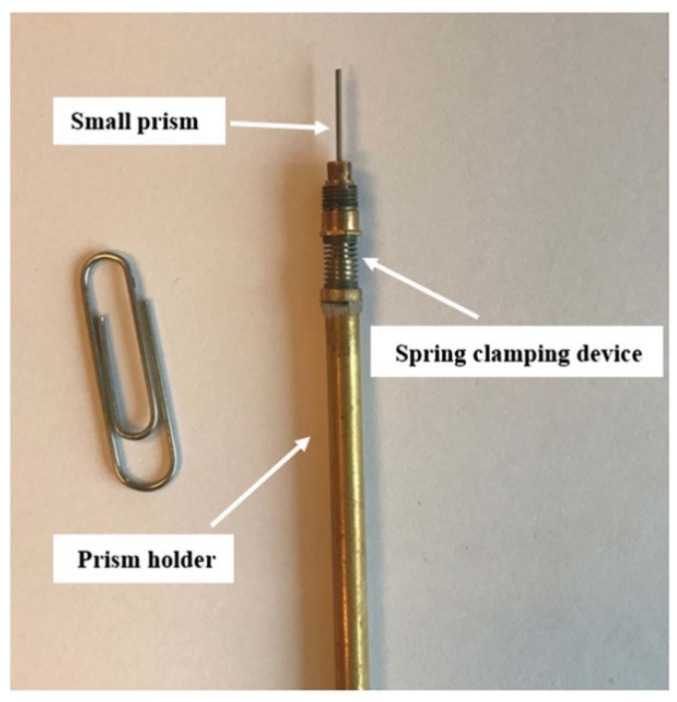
A micro-beam clamped on a custom-made holder, prior to X-ray CT scanning [29].
